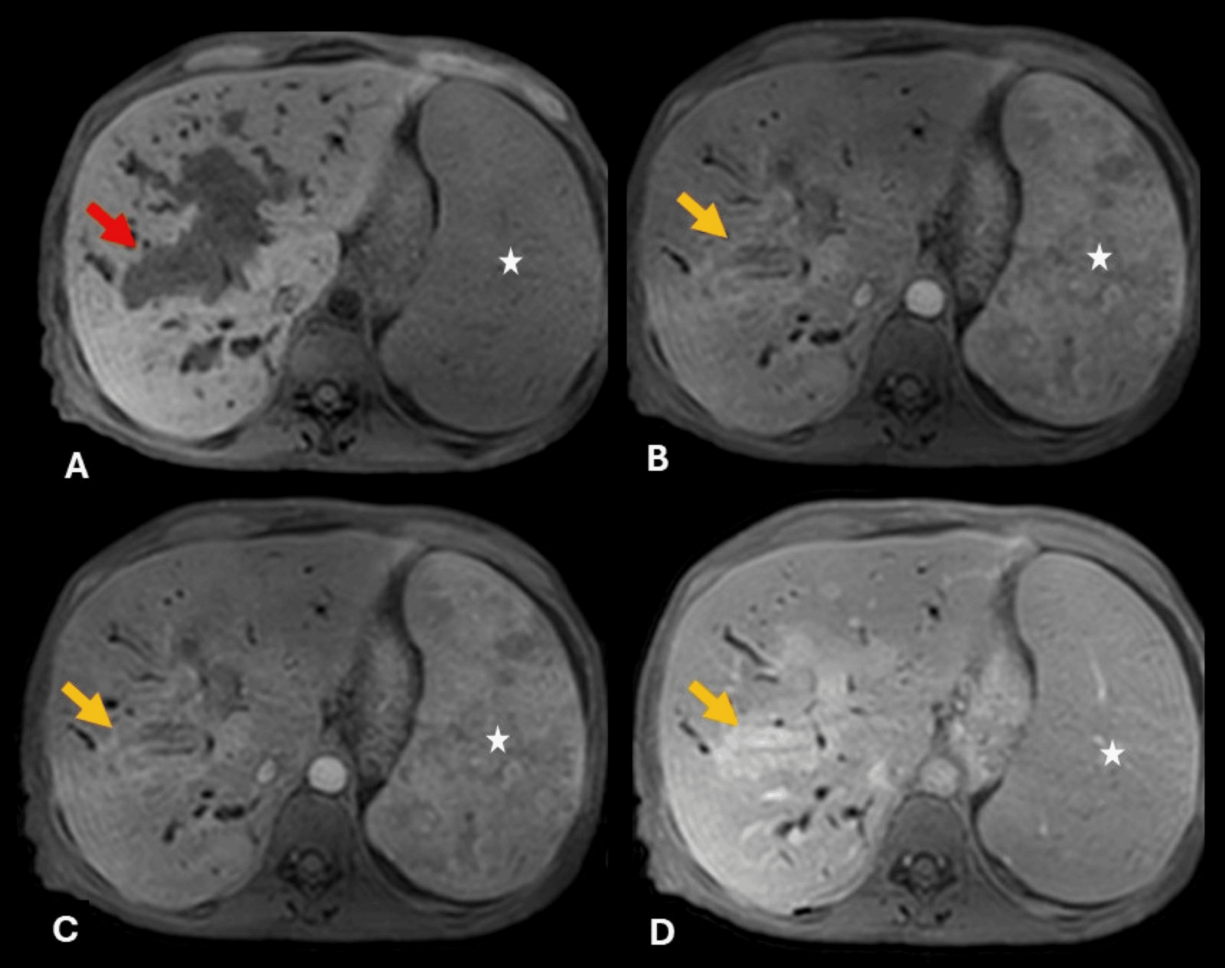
Contrast-enhanced MRI with dynamic T1 sequences reveals nodular periportal soft tissue, hypointense in T1 (red arrow in A), with mild enhancement during the arterial phase that persists without increasing in subsequent phases (yellow arrows). Splenomegaly and heterogeneous splenic enhancement (white star) are also present.
